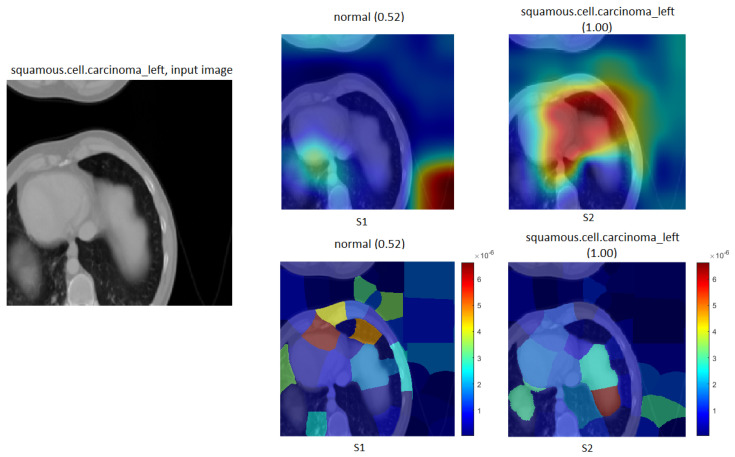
Grad-CAM in the first row and LIME in the second row for chest CT scan images. The correct classification is squamous.cell.carcinoma-left.
